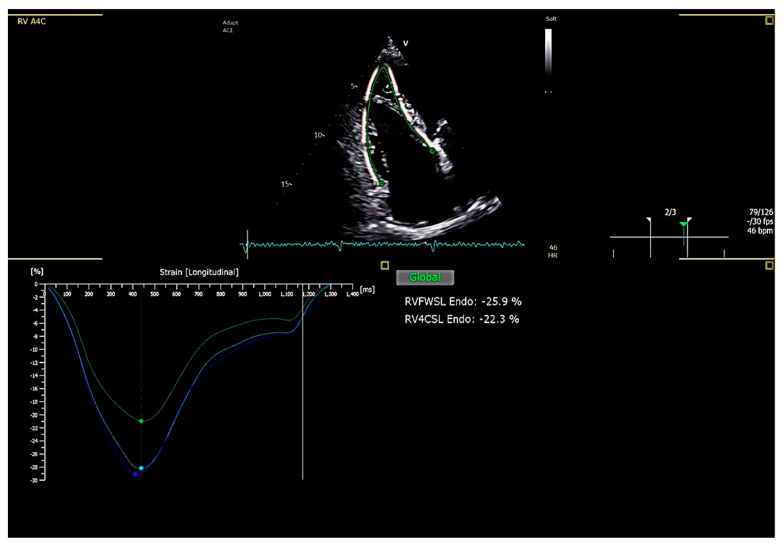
Right Ventricular Free Wall Longitudinal Strain (assessed using speckle-tracking echocardiography). RVFWLS = Right Ventricular Free Wall Longitudinal Strain; RV4CLS = Right Ventricular 4-Chamber Longitudinal Strain.
